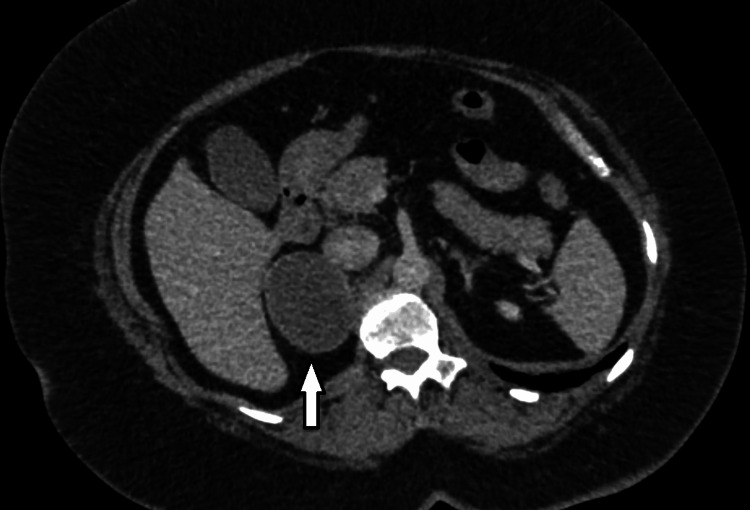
CT abdomen with contrast (case 2) - large right-sided cystic mass (arrow) seen below the liver but above the level of the kidney. CT: computed tomography
